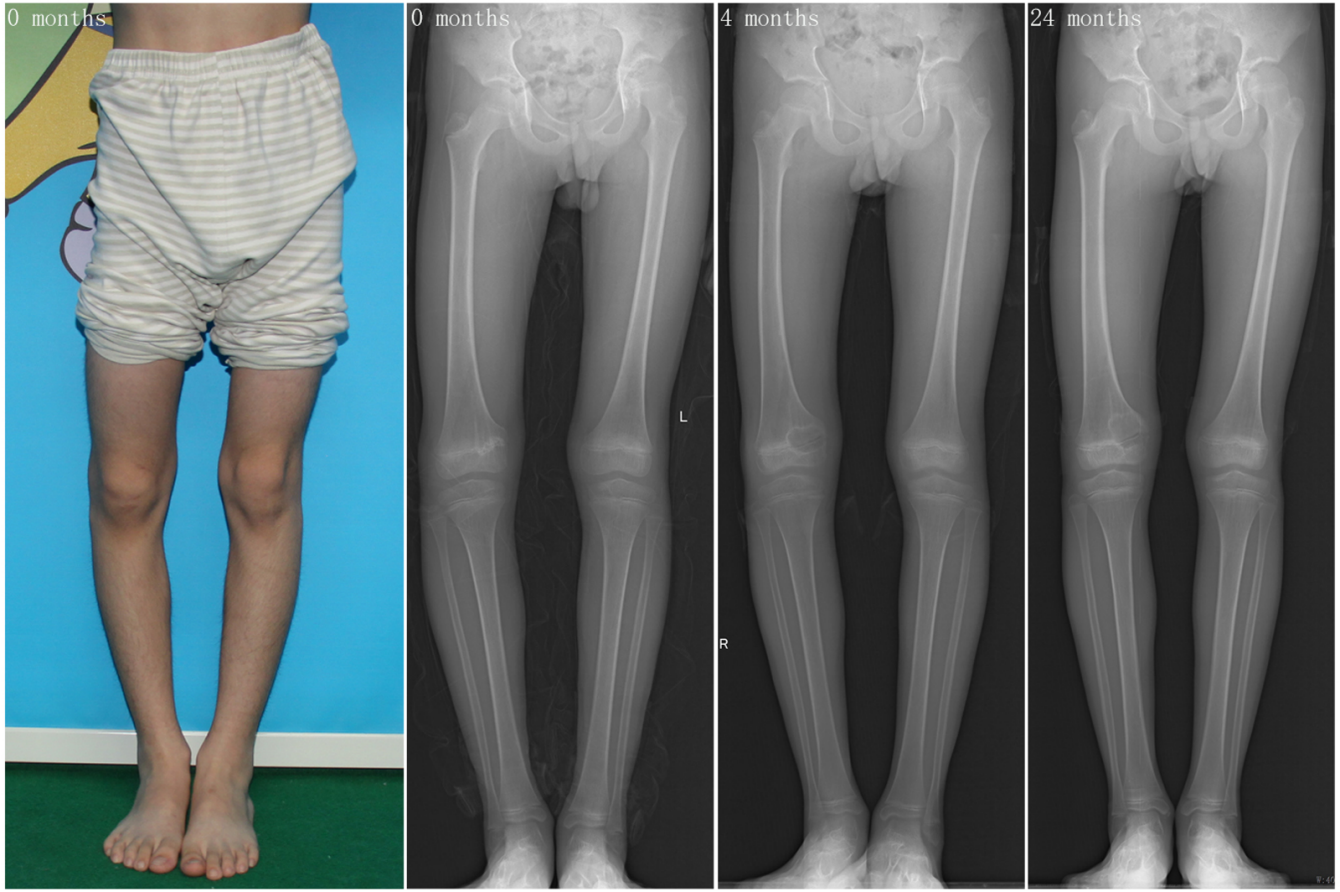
Ten years old boy who found progressively varus deformity of the right knee joint about one year (case 2): the gross view, anterior-posterior radiograph of the patient.
